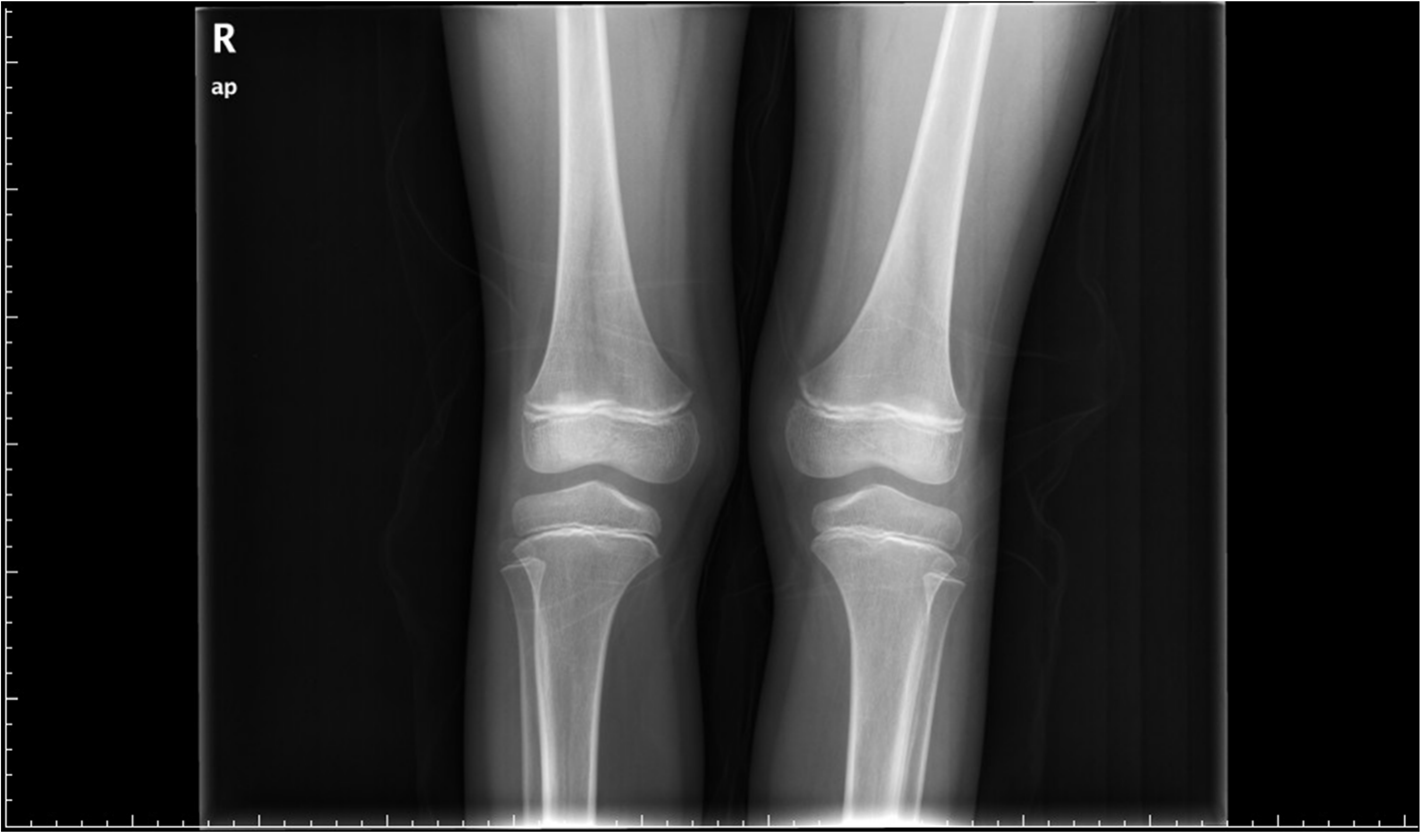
Anteroposterior radiograph of the knees bilaterally
